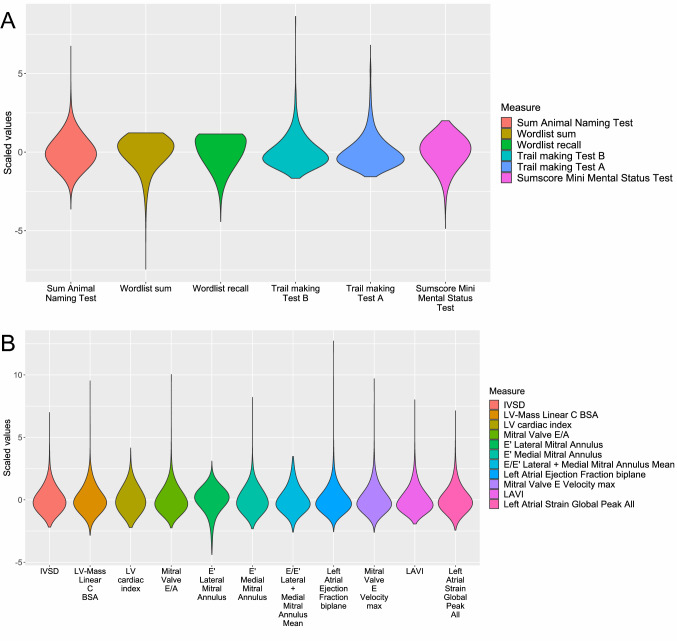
Violin plots showing the distribution of cognitive function test results (Panel A) and echocardiographic measures (Panel B) in the cohort. *BSA* body surface area; *IVSD* interventricular septal thickness; *LAVI* left atrial volume index; *LV* left ventricular
